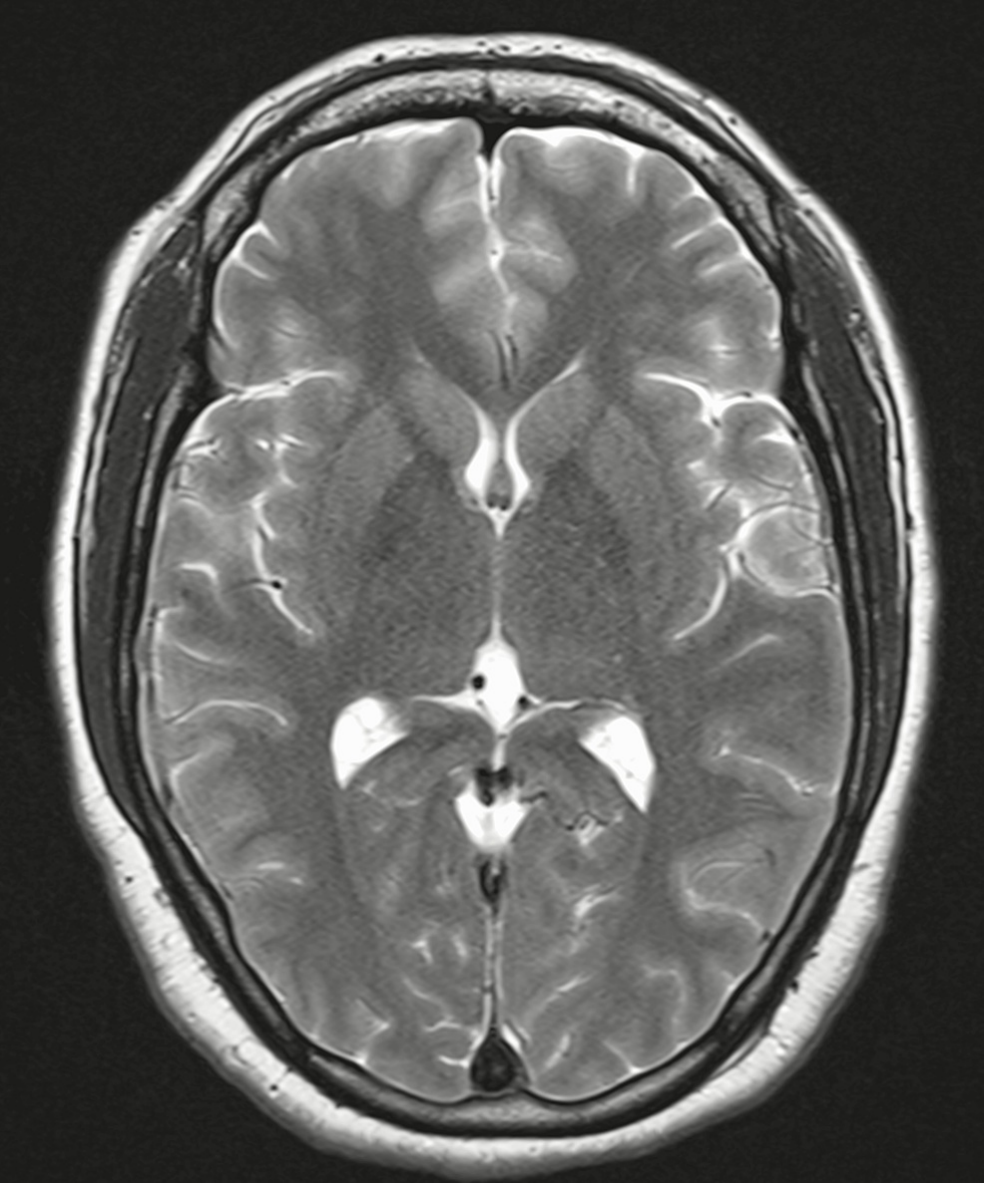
Contrast Enhanced MRI Brain - Cerebral Venous Sinus Thrombosis A non-invasive diagnostic procedure that uses a combination of a large magnet, radio frequencies, and a computer to produce detailed images of organs and structures within the body without the use of damaging ionizing radiation. There are filling defects within the superior sagittal sinus, left transverse & sigmoid sinuses, and the left jugular bulb. Filling defects are also seen in the right transverse and straight sinus and the Galen region's vein. Cortical veins draining into these sinuses appear engorged, suggesting thrombosis.
